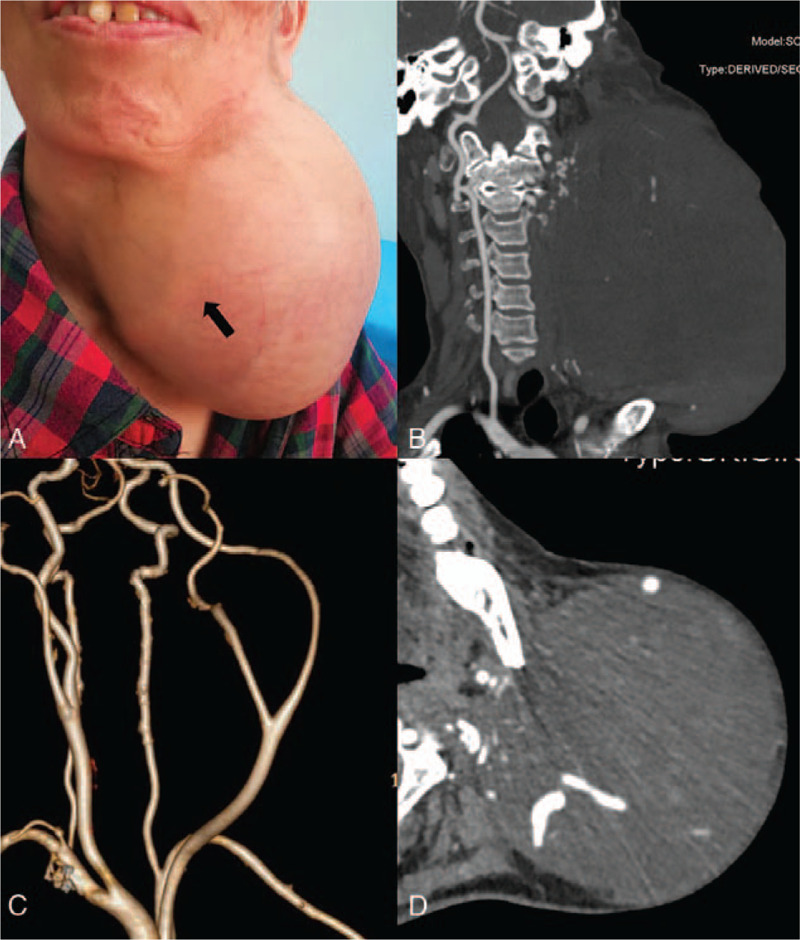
(A) Appearance of the front view of the mass in the neck, with “Y” shape carotid artery bifurcation visible (arrow). (B) Coronal view of the computed tomography angiography (CTA) of the patient. (C) CTA showed carotid arteries were compressed and translocated by the mass. (D) Axial view of CTA revealed the left carotid artery was involved by the mass.
